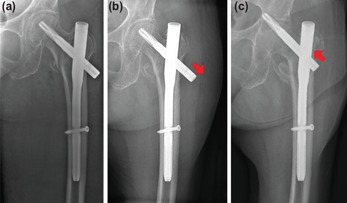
Plain radiographs post fixation of the pertrochanteric left femur fracture with a cephalomedullary device (Synthes proximal femur antirotation nail, PFNA) (a) Immediate postop (b) Subsequent impaction of the fracture with gradual lateral migration of the femoral neck element with respect to the intramedullary nail component (red arrow) (c) Medial migration of the femoral neck element with respect to the intramedullary nail component with perforation of the femoral head and penetration of the acetabulum (red arrow).
